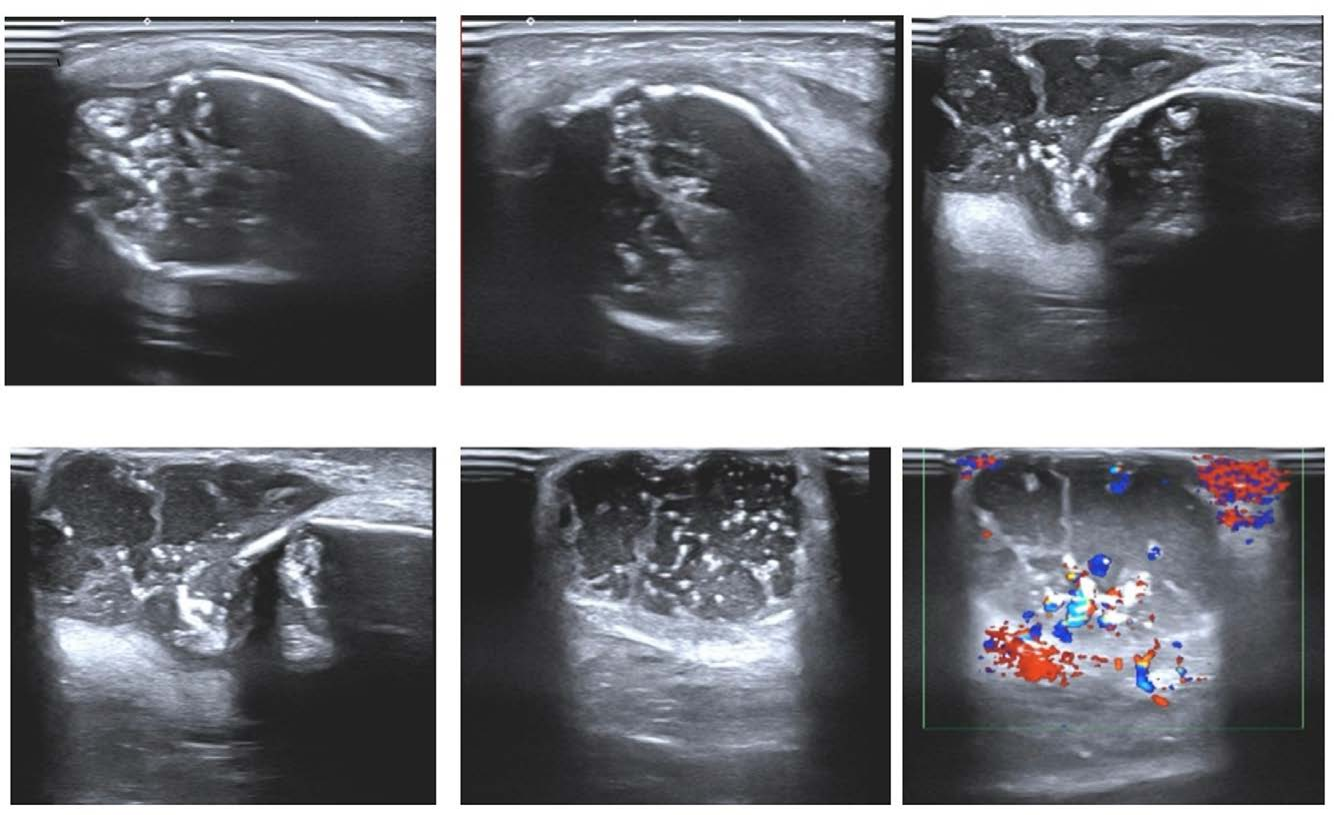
The right ulna underwent expansive changes, characterized by thinning of the lateral cortex, disrupted echogenicity, and extension into soft tissue, resulting in a cystic mass with mixed echogenicity in the soft tissue layer, measuring approximately 36 × 22 mm, surrounded by significant blood flow signals on color Doppler flow imaging (CDFI).
